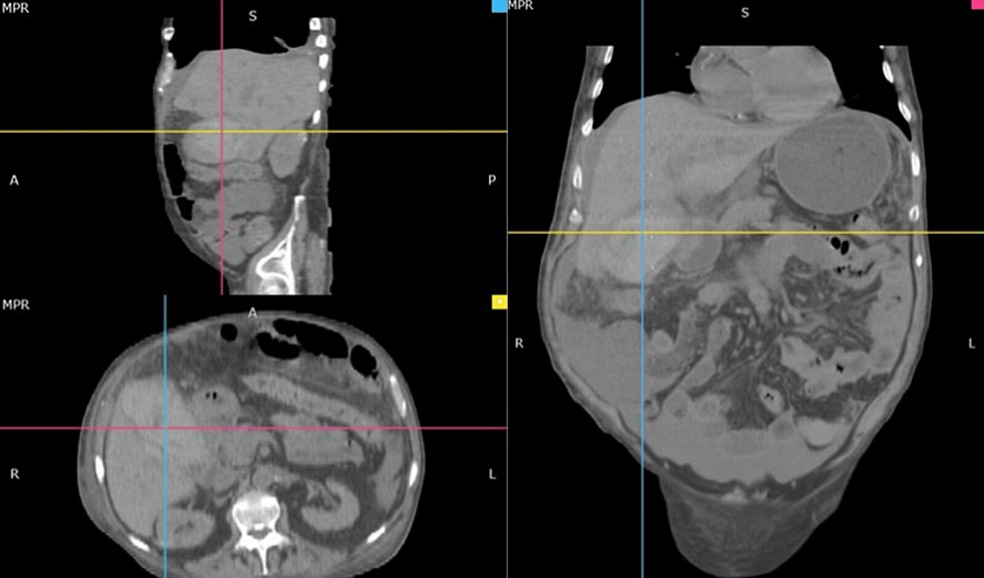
CT of the abdomen and pelvis without contrast. Perforated gallbladder with hematic content.
